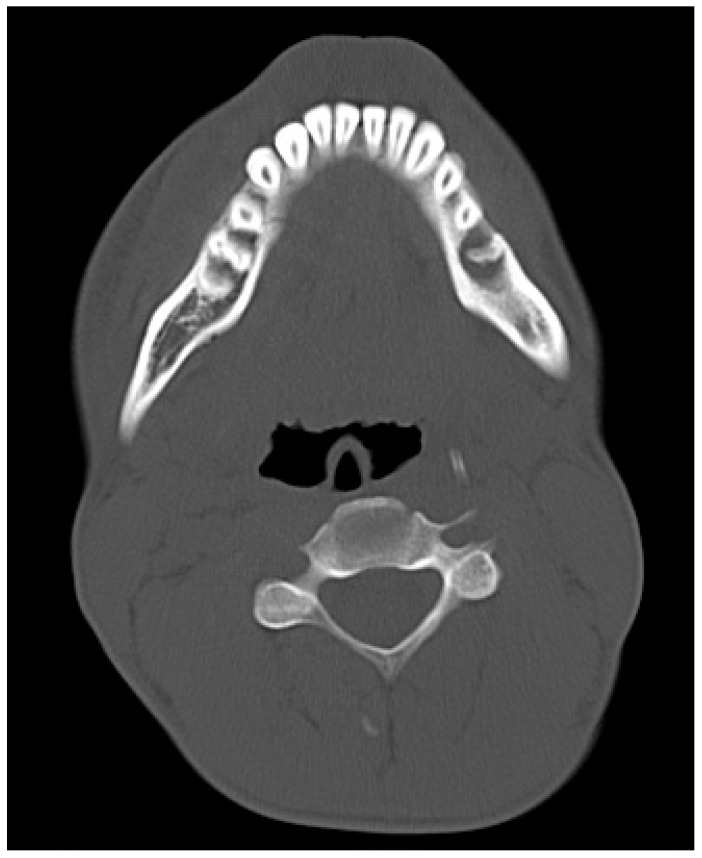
Computed tomography in axial plane showing the interrupted continuity of the bone structure near tooth 45.
